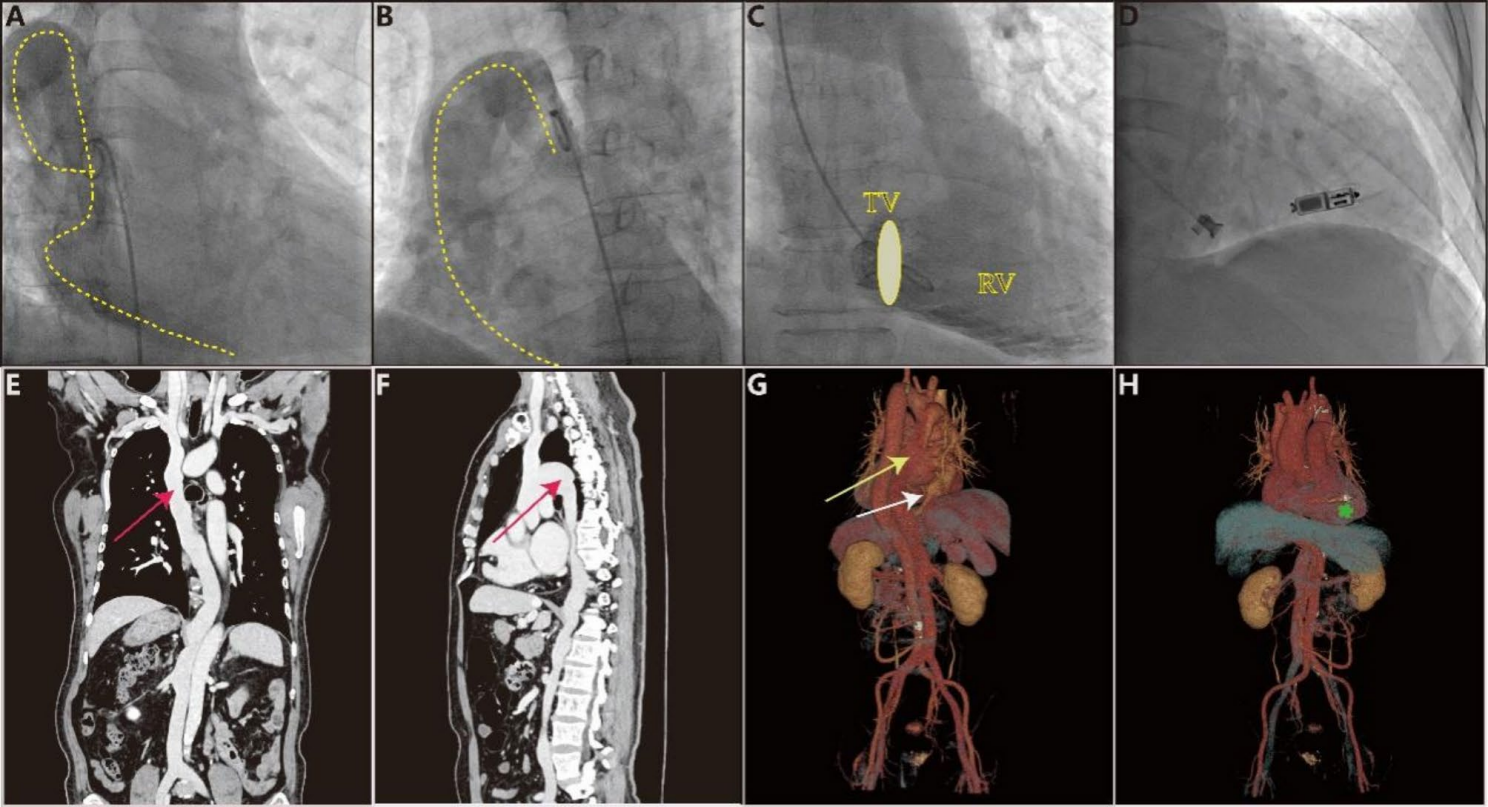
Angiography and CT imaging of the congenital venous anatomy variant, and leadless pacemaker anchored position. A, B) non-selective venous angiography in RAO and LAO view via femoral vein. The yellow dotted line shows the tortuous and compensatory enlarged azygos vein drainage into RA directly. C) non-selective venous angiography of RV via right jugular vein. The yellow oval showed the tricuspid valve (TV). D). the leadless pacemaker anchored in RV septal via jugular vein. E, F) the post-procedural CT imaging of the venous angiography in anteroposterior (AP) and lateral view. The red arrow showed the abnormally tortuous and enlarged azygos. G) the 3-D image reconstruction. The yellow arrow showed the abnormally tortuous and enlarged azygos. The white arrow showed the hepatic vein directly drained into RA. H) the 3-D image reconstruction in AP view. The green asterisk showed the leadless pacemaker
